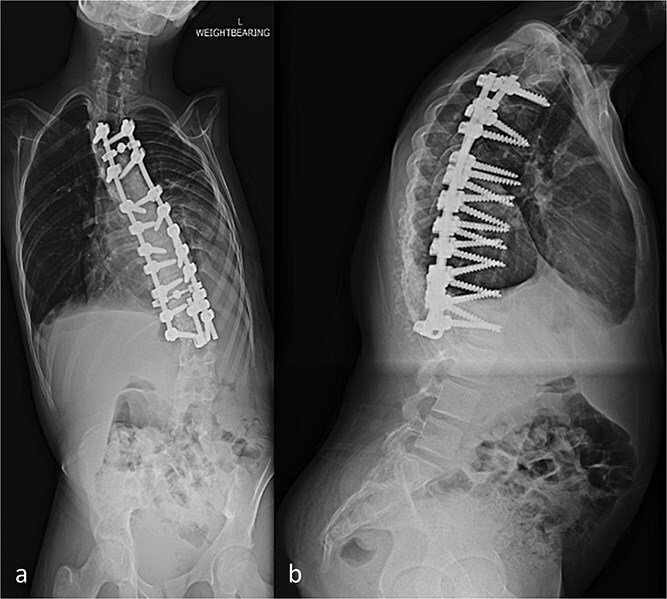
Postoperative whole spine radiographs. AP (a) and lateral (b) views showing improved alignment with Cobb angle reduced to 34° after selective thoracic fusion.
